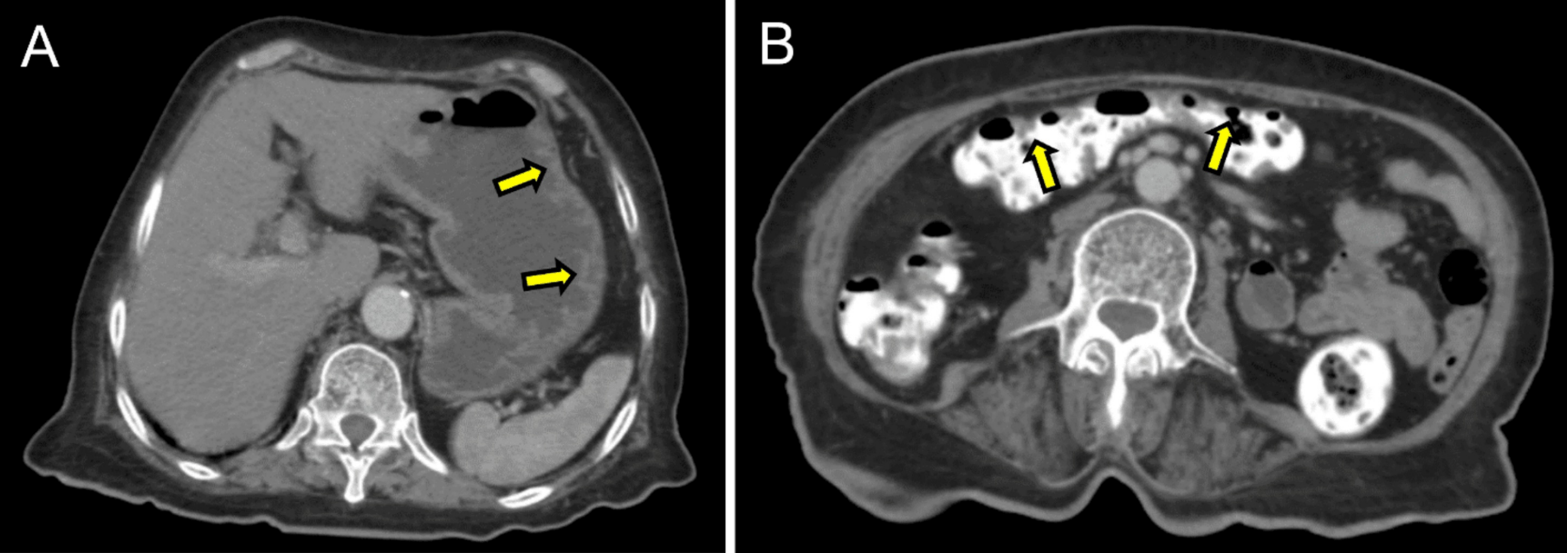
Abdominal axial CT Irregular thickening of the gastric wall (arrows) (A) and the colon (arrows) (B) are noted.
